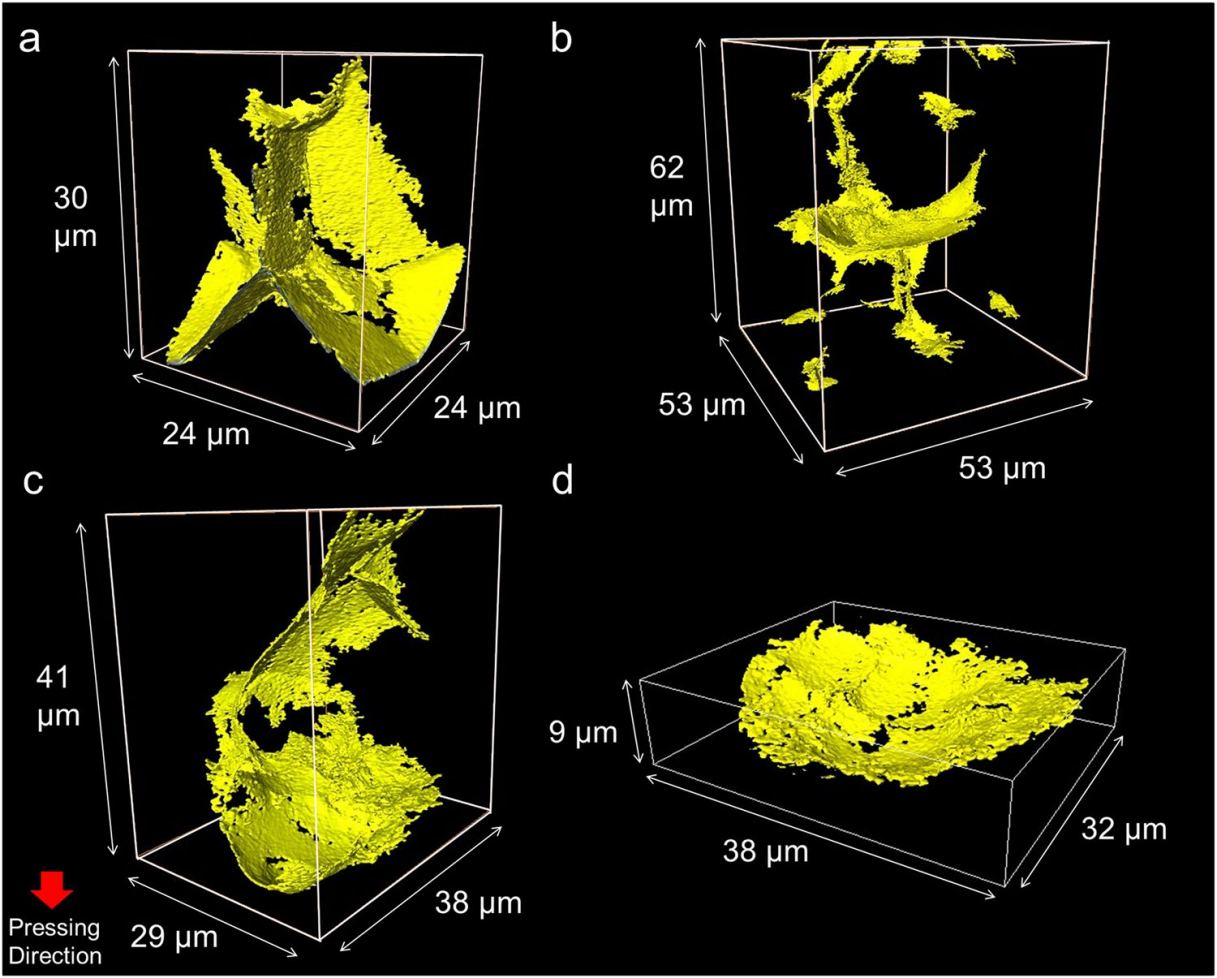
Nano-CT images of defects. (a–c) Branched crack-like defects (Type II), (d) shallow bowl-shaped crack (Type III).
